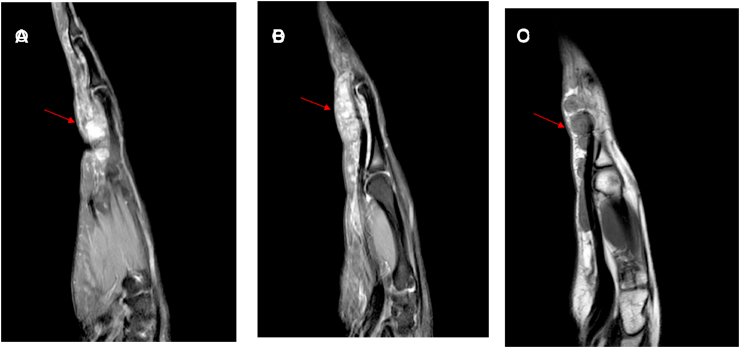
(A,B,C) MRI showed these masses on the surface of the flexor tendens.
